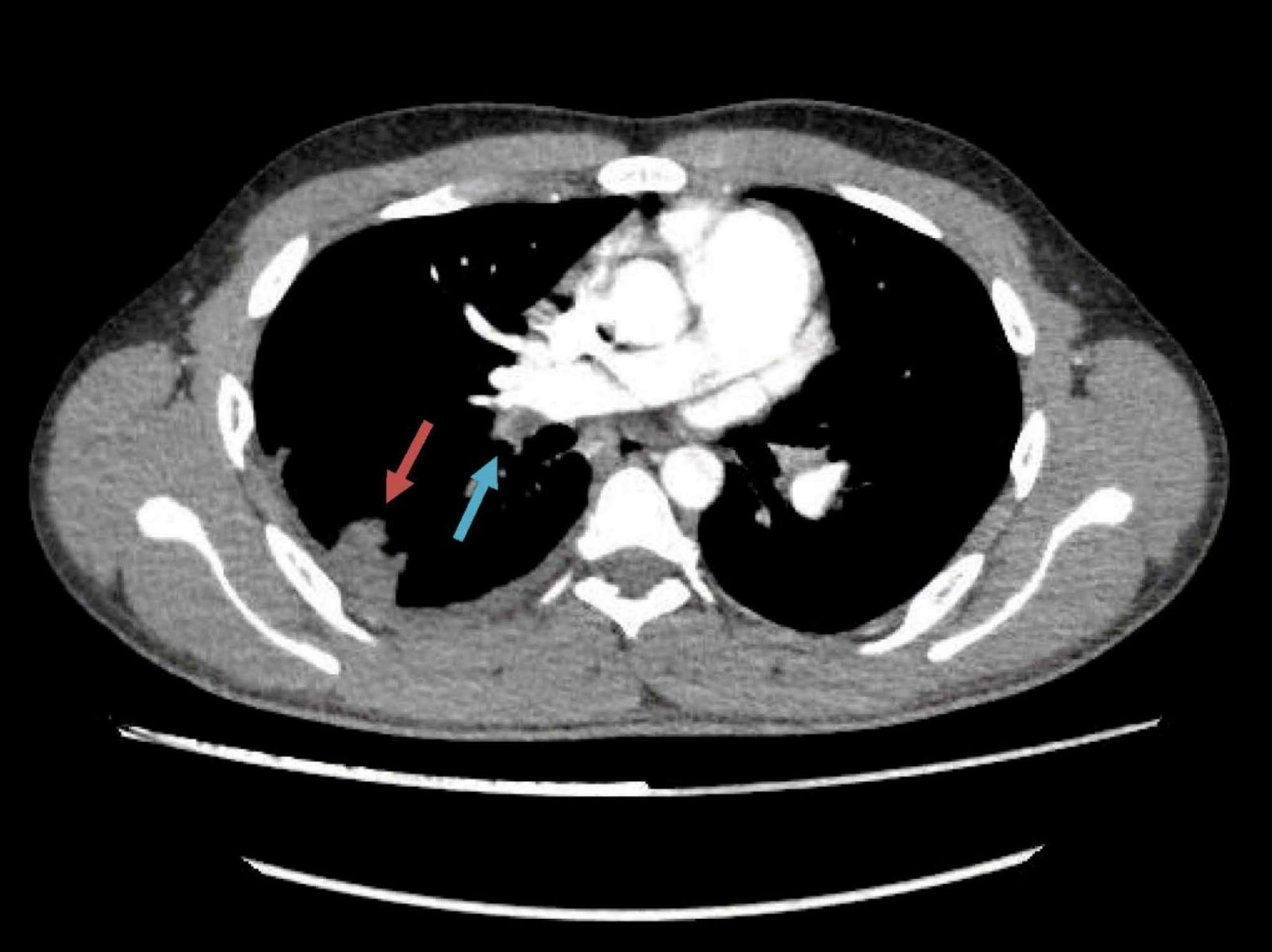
Contrast-enhanced computed tomography pulmonary angiogram. Blue arrow shows a filling defect in distal most right main pulmonary artery suggestive of pulmonary embolism. Red arrow shows wedge shape area of dense consolidation in right lateral basal segment representing infarct.
